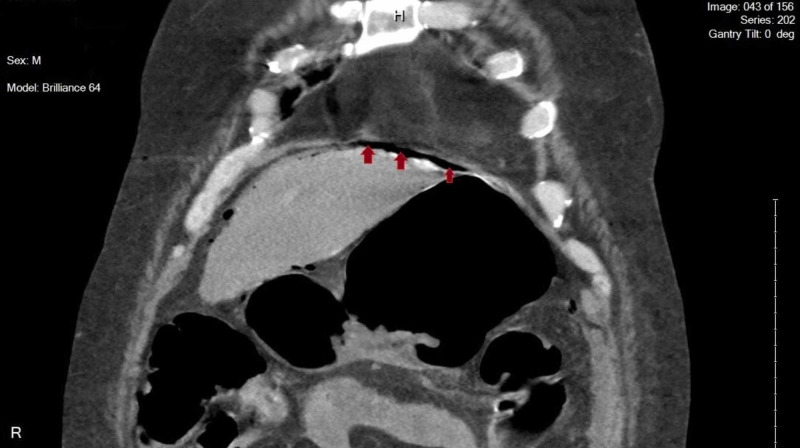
CT abdomen/pelvis demonstrating pneumoperitoneum
